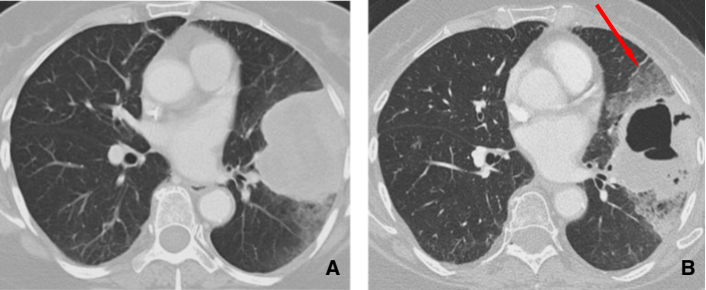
71 year old female with NSCLC on an erlotinib. A: Initial CT shows a large and lobulated left upper lobe mass extending to the pleural surface. B: CT scan obtained 2 months later demonstrates cavitation within the central portion of the mass with development of surrounding ground glass opacities secondary to intralveolar hemorrhage (arrow).
